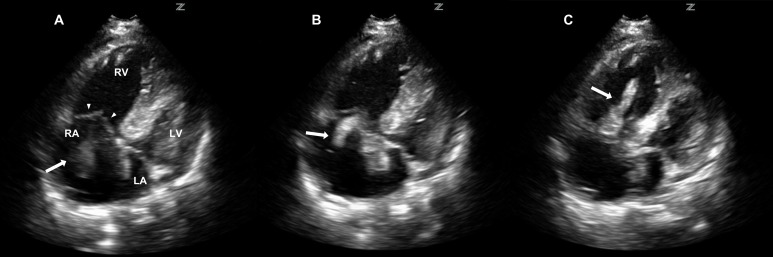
Bedside echocardiography in the four chamber view shows a free-floating atrial clot (arrow) as it glides between the right atrium (RA) and moves to the right ventricle (RV) during cardiac diastole and systole. *A*, the clot (arrow) is seen starting in the RA, *B,* moving through tricuspid valve (arrowheads seen in A), *C,* and finally seen in the RV. *LA*, left atrium. *LV*, left ventricle
